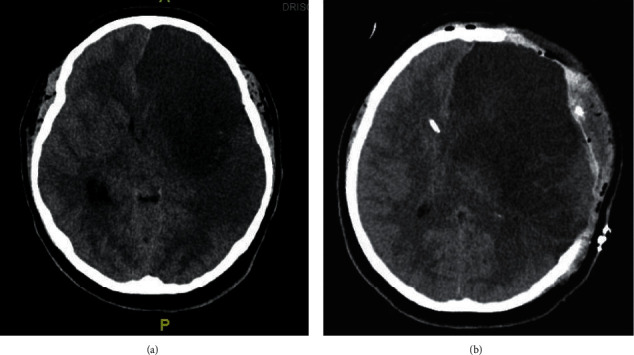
(a) : CT scan head WO contrast. A large hypodensity of the left MCA territory, predominantly involving the left frontal lobe, basal ganglia, insula, and left temporoparietal lobes; worsened since the prior MRI study. There is a significant mass effect on the left lateral ventricle. There is an interval development of uncal herniation and anterior subfalcine measuring 7-8 mm towards the right. There is an obliteration of the suprasellar and perimesencephalic cisterns. (b) CT Scan head WO contrast.
